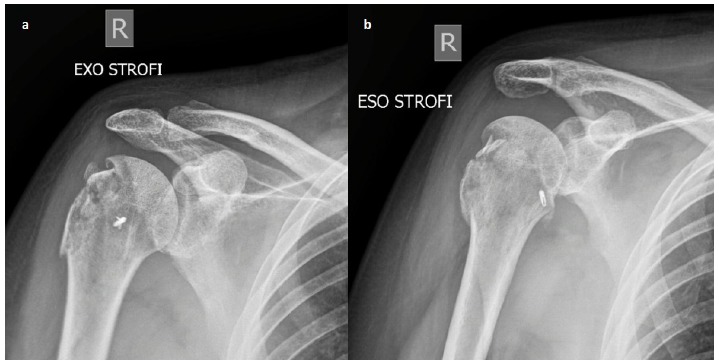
Follow up anteroposterior x-ray of the right shoulder in external (a) internal (b) and rotation showing a stable joint and good healing of the greater tuberosity.
